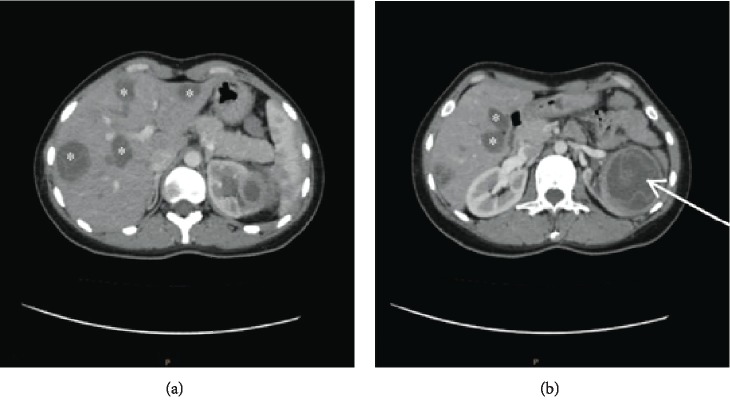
Abdominal CT scan of the patient showing (a) multiple cysts in the liver “∗” and (b) multiple cysts in the liver “∗” and a solitary cyst in the kidney “arrow”.
